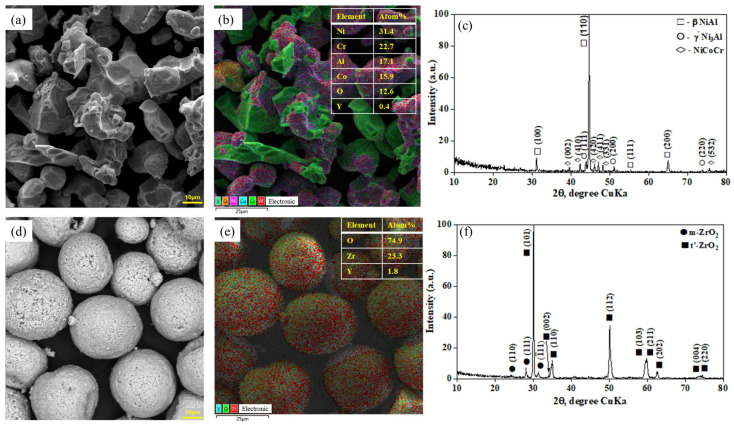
Microstructure of the powders and results of X-ray phase analysis of phase composition: (a–c) NiCrAlY powder; (d–f) YSZ powder.
